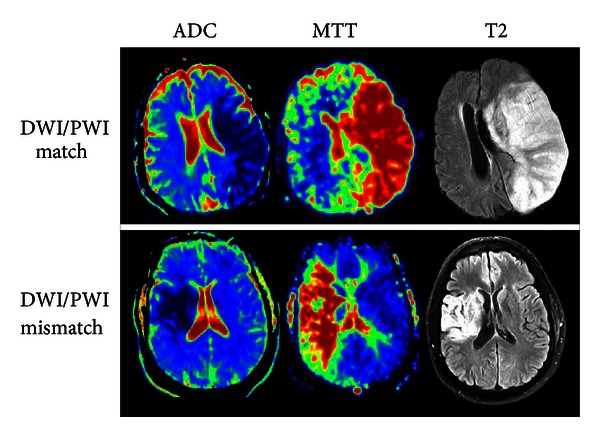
ADC and MTT maps demonstrating DWI/PWI match and mismatch in relation to the MRI T2 lesion.
